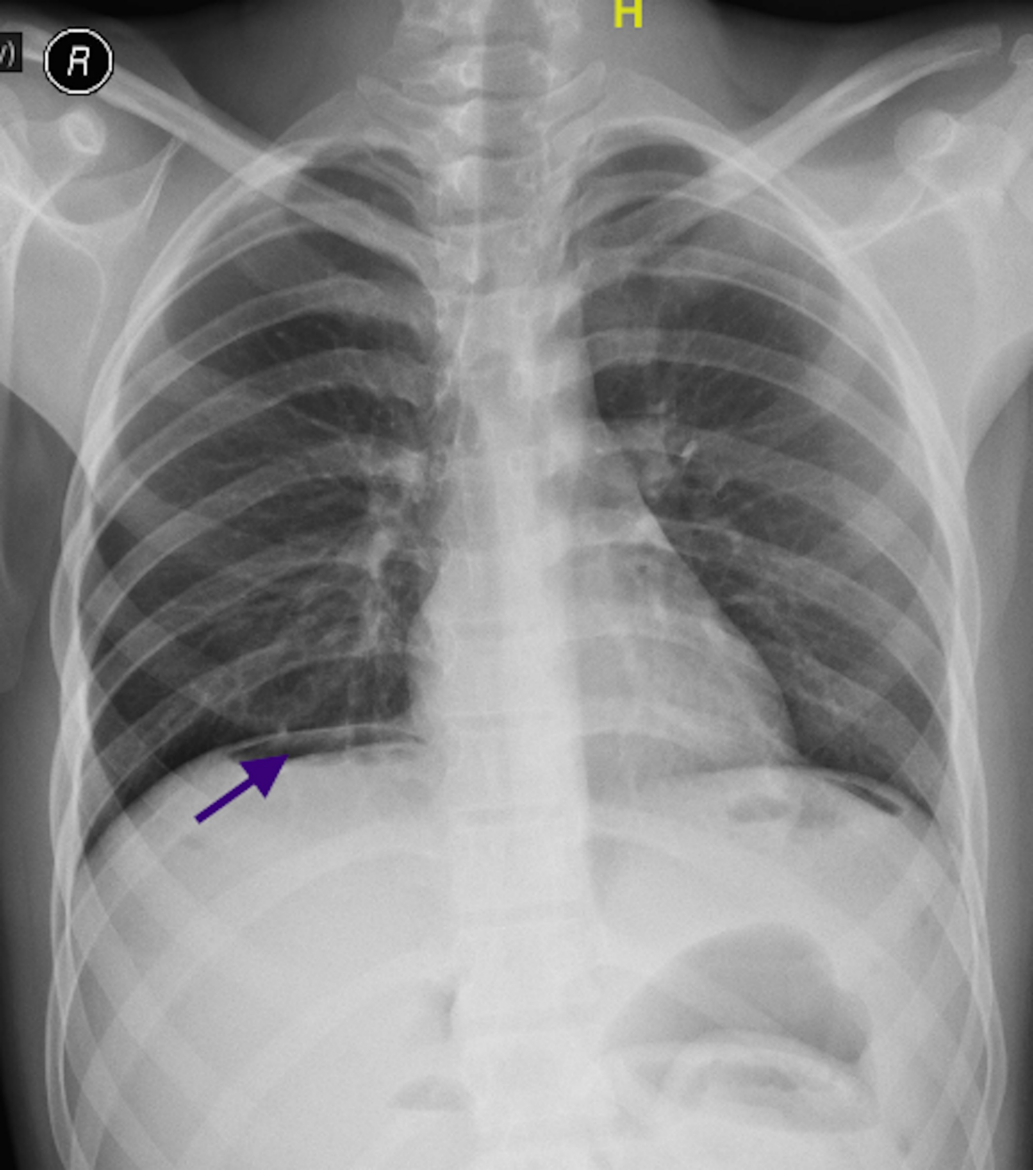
Chest X-ray erect view shows air under diaphragm (marked by arrow)
